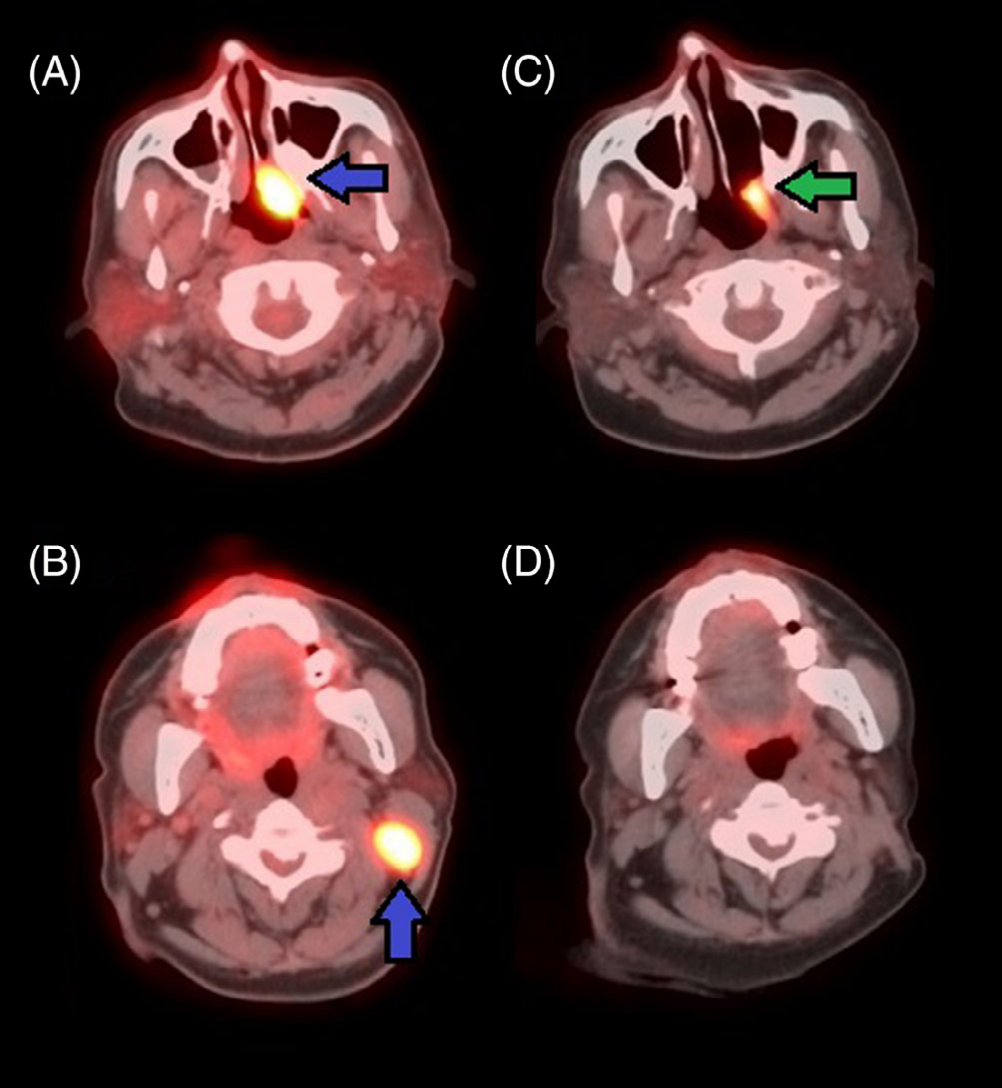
(A, B) PET/CT scan in February 2020: Hypermetabolic left posterior nasal cavity mass consistent with patient's known malignant tumor measuring 1.5 × 2.7 cm demonstrating a maximum SUV of 16.2 (blue, left arrow). Enlarged hypermetabolic left cervical level IIb lymph node consistent with regional metastasis measuring 1.8 × 2.2 cm with a maximum SUV of 15.6 (blue, up arrow). (C, D) PET/CT scan in October 2021: Hypermetabolic triangular shaped mass in the left posterior sidewall of the nasopharynx that appears to be decreased in size when compared to prior study; mass measures 1.1 × 2.2 cm with a maximum SUV of 8.9 (green, left arrow). Enlarged hypermetabolic left cervical level IIb lymph node identified on previous study is no longer present.
